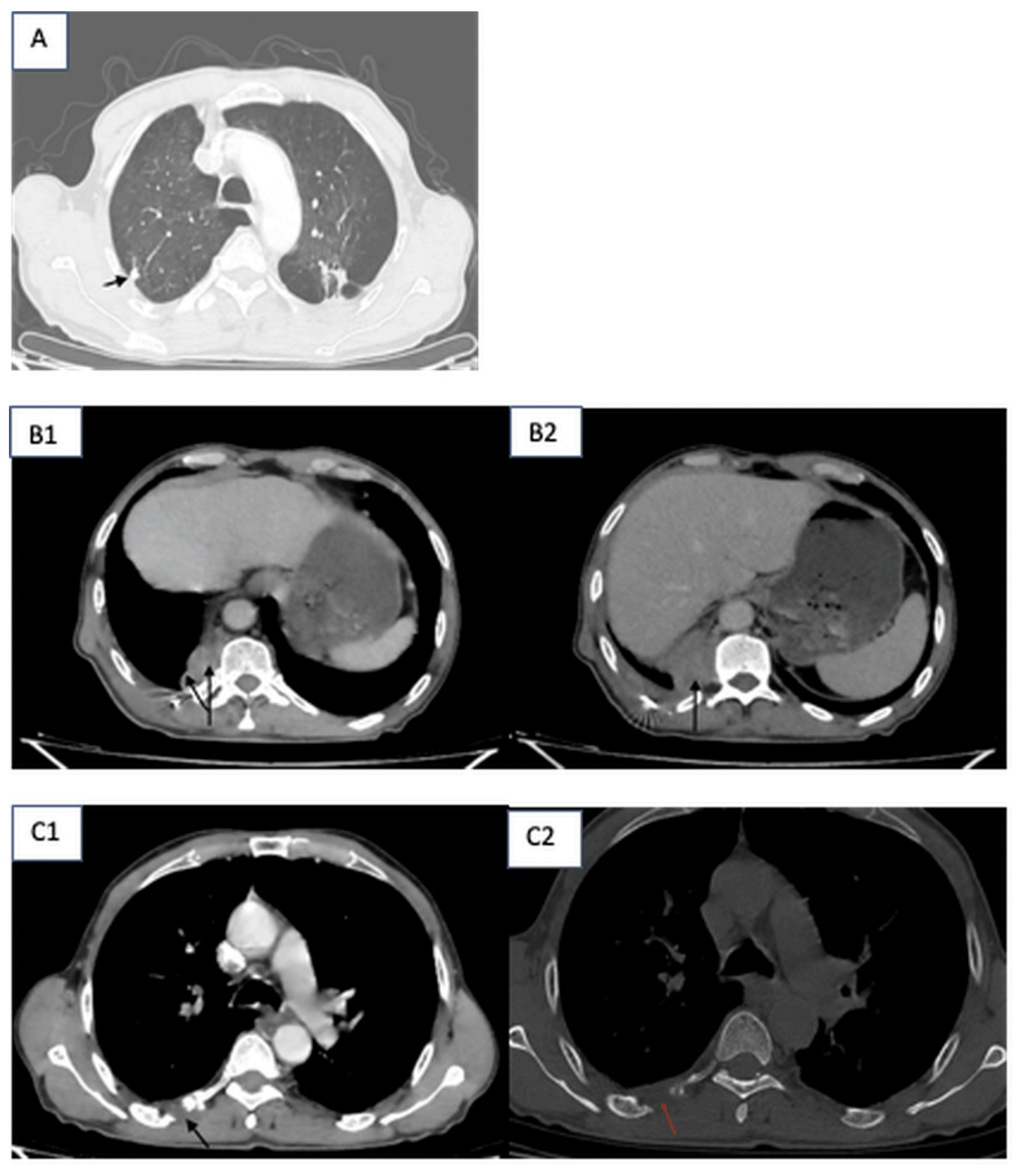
Post-operative CT scan in parenchymal, mediastinal, and bone windows. (A) Axial CT scan in parenchymal window showing a metastatic subpleural node measuring 15.9x16.5 mm. (B1) Axial CT scan in mediastinal window showing a right dorsobasal mass, with heterogeneous enhancement, and metastatic pleural nodes (B2), the largest measuring 13.9x19.5 mm. (C1) and (C2) Axial CT scan in bone and mediastinal windows showing metastasis involving the posterior arch of fifth right rib.
